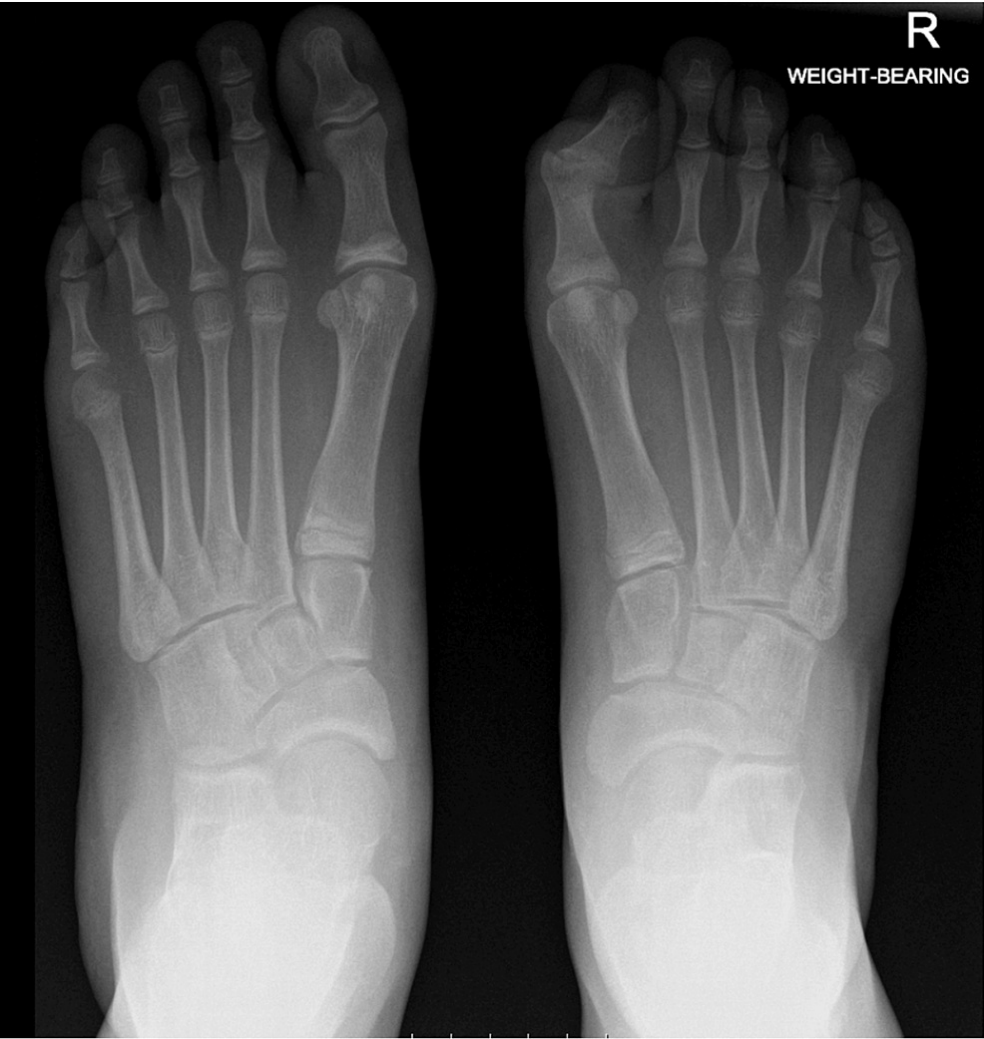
AP weight-bearing X-rays of both feet. The IPJ of the right hallux appears flexed and valgus angulated, but the X-rays are otherwise unremarkable AP, anteroposterior
